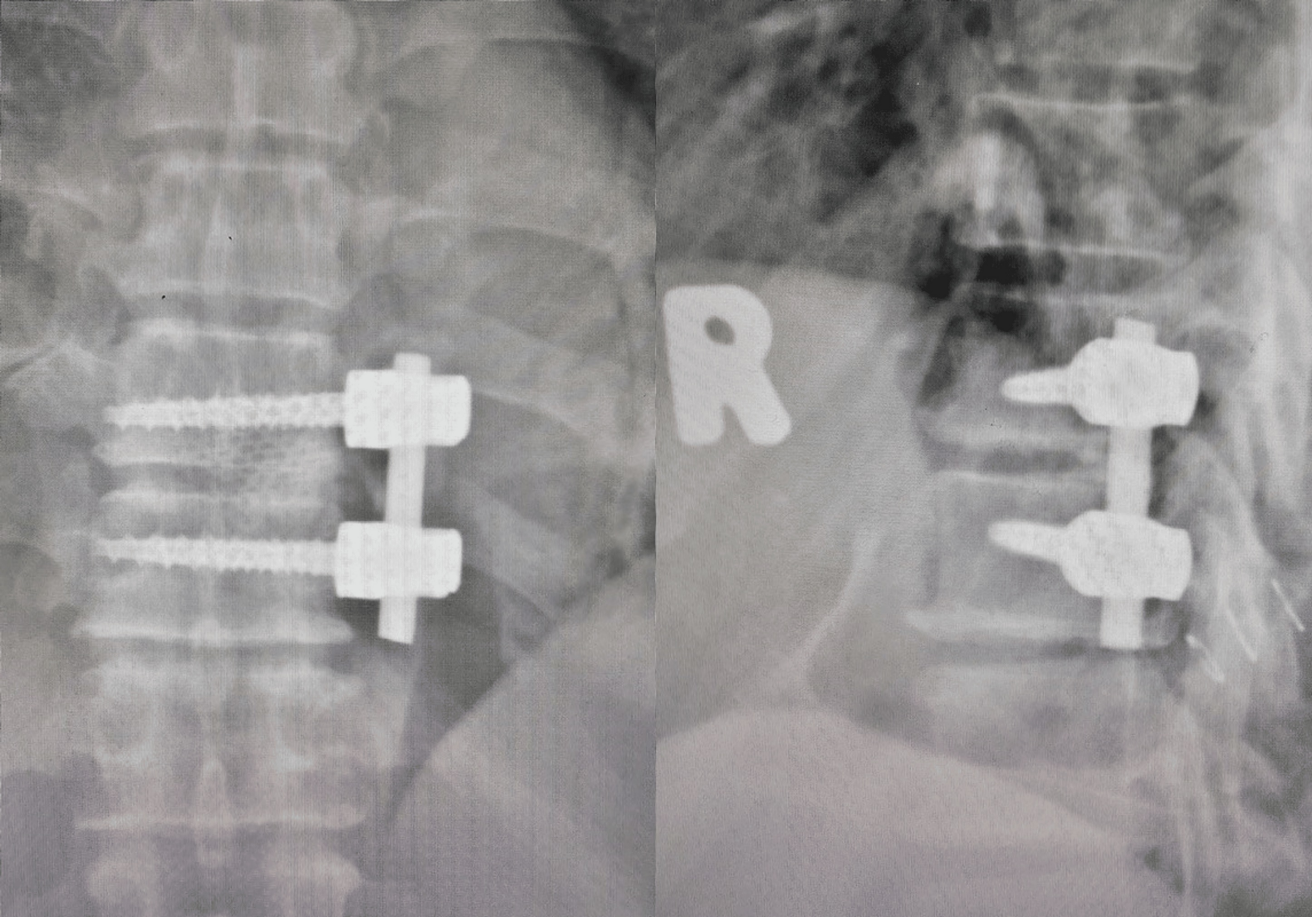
Postoperative plain radiograph showed the implant in situ.
